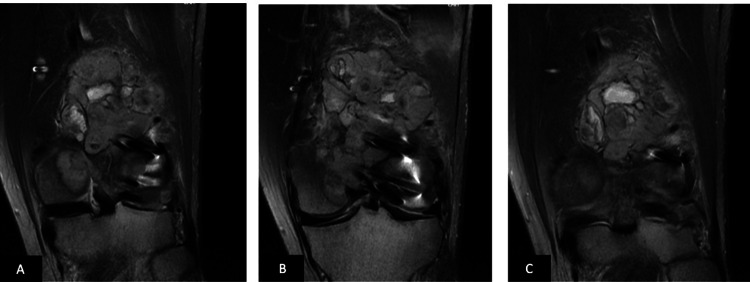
Magnetic resonance images of the patient. (A-B-C) Coronal views of the patient.
